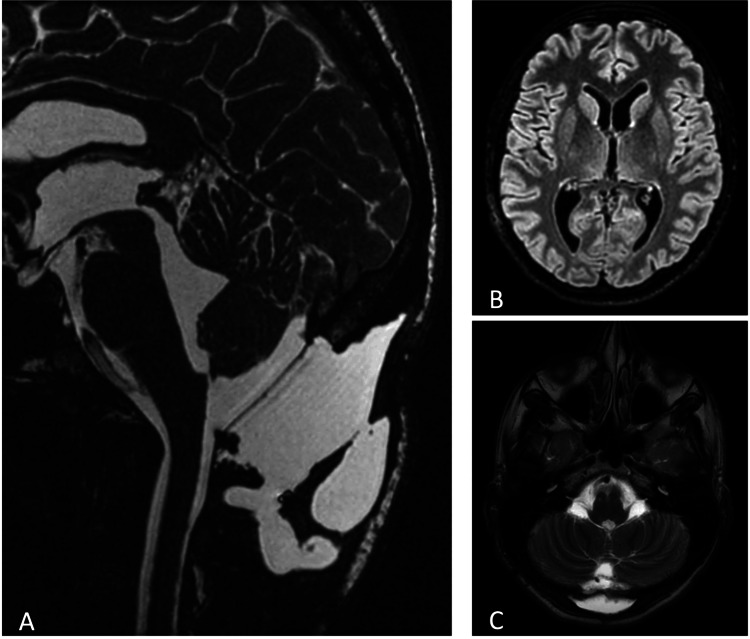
MRI at 1.5 months after membrane fenestration with no obstruction of the foramen of Magendie or signs of hydrocephalus. A: Both the fourth ventricle and floor of the third ventricle look normal. B: Supratentorial ventricles are normal, with no transependymal oedema. C: Some dilatation of the Luschka foramina is still observed. A slight enlargement of the pseudomeningocele is noted.
